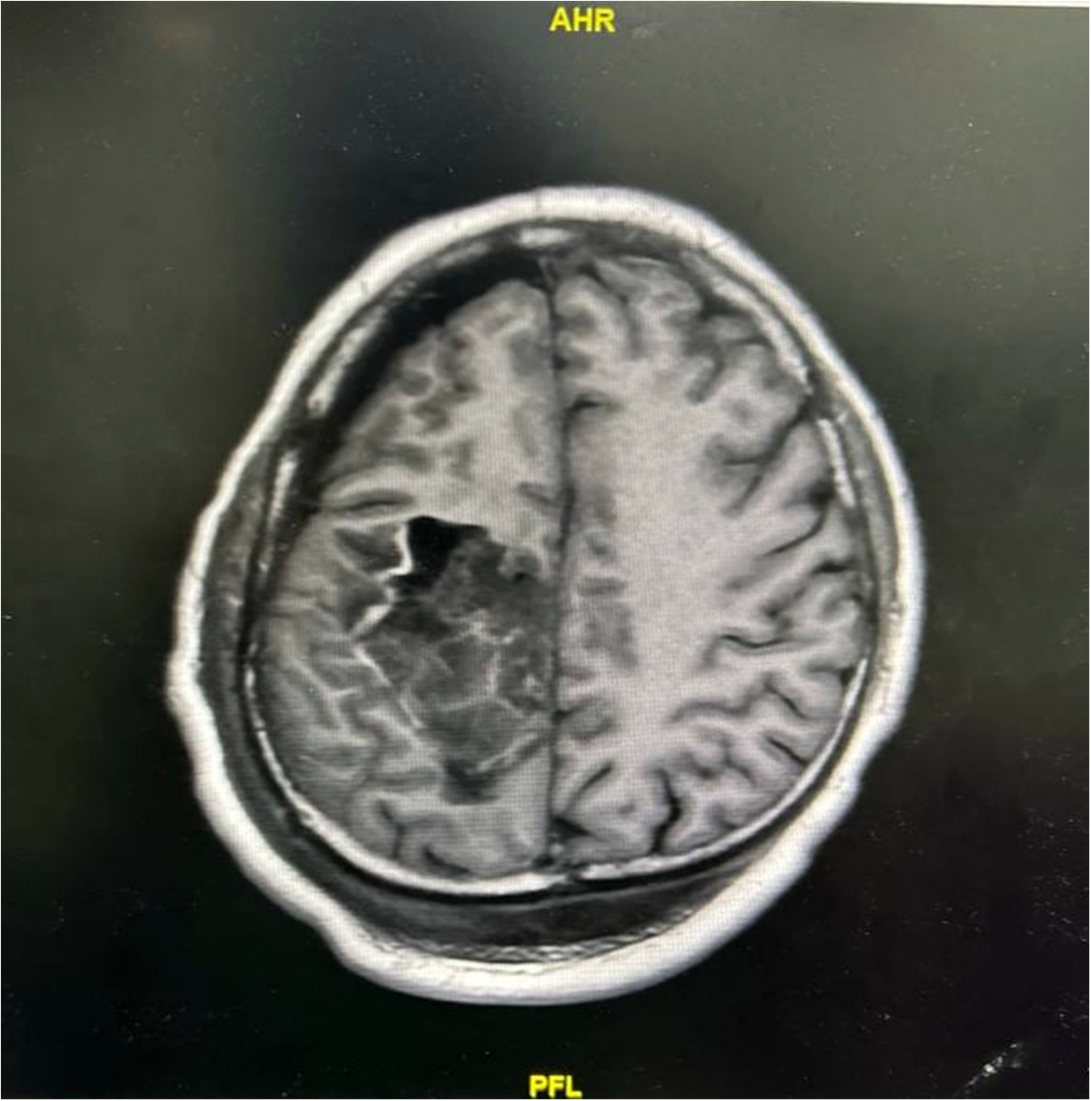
Magnetic resonance imaging of the brain showing a well‐defined, lobulated, enhancing lesion in the right precentral gyrus with mild perilesional oedema.
